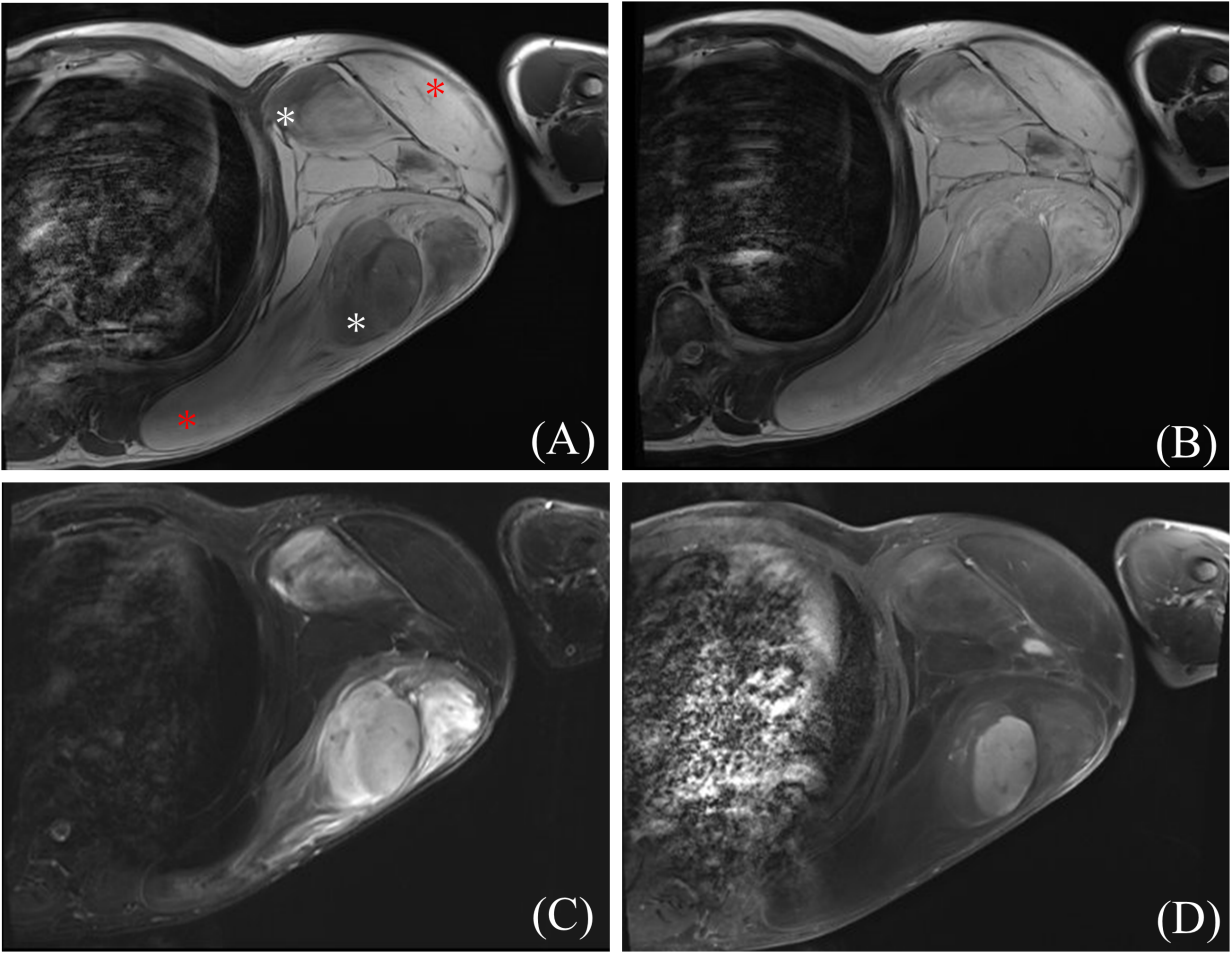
Representative magnetic resonance images of dedifferentiated liposarcoma (DDLPS). (A) T1-weighted image (white asterisk indicates DDLPS and red asterisk indicates well-differentiated liposarcoma components), (B) T2-weighted image, (C) short tau inversion recovery image, and (D) gadolinium-enhanced T1-weighted image.
